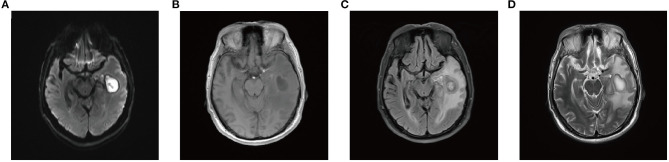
Brain MRI 10 days after mNGS. (A) DWI; (B) T1WI; (C) T2-FLAIR; (D) T2WI. MRI, magnetic resonance imaging; mNGS, metagenomic next-generation sequencing; DWI, diffusion-weighted imaging; T1WI, T1-weighted imaging; FLAIR, fluid-attenuated inversion recovery; T2WI, T2-weighted imaging.
